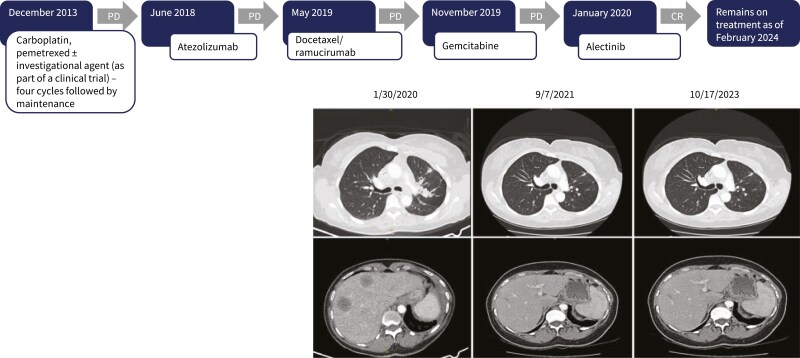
The case is of a 62-year-old female from Trinidad, never smoker who presented with shortness of breath in December 2013. She underwent thoracentesis (left pleural tap) that confirmed adenocarcinoma (thyroid transcription factor-1, TTF-1, +ve). Molecular testing returned negative results for *EGFR*-mutated, *KRAS* wild type, and ALK fusions (the latter performed using FISH). She entered a clinical trial and received four cycles of standard of care plus the investigational agent followed by maintenance, which she continued receiving until June 2018. In the subsequent years, she progressed under other therapies as indicated and in January 2020 her performance status started to decline. At the time, the treatment options considered were palliative care (hospice), another chemotherapy (eg, nab-paclitaxel, irinotecan), or a clinical trial. To aid decision, liquid biopsy for NGS analysis was also performed (preferably chosen over tissue re-biopsy). Plasma-based NGS this time revealed ALK mutation (EML4-ALK fusion) and in February 2020 treatment with alectinib was initiated. The patient’s performance status improved, and she showed complete response (CR) to treatment on follow-up CT scans. She experienced no significant toxicity from alectinib and remains on treatment as of February 2024. Provided courtesy of Dr Tarek Mekhail, AdventHealth Cancer Institute, Florida, USA.
